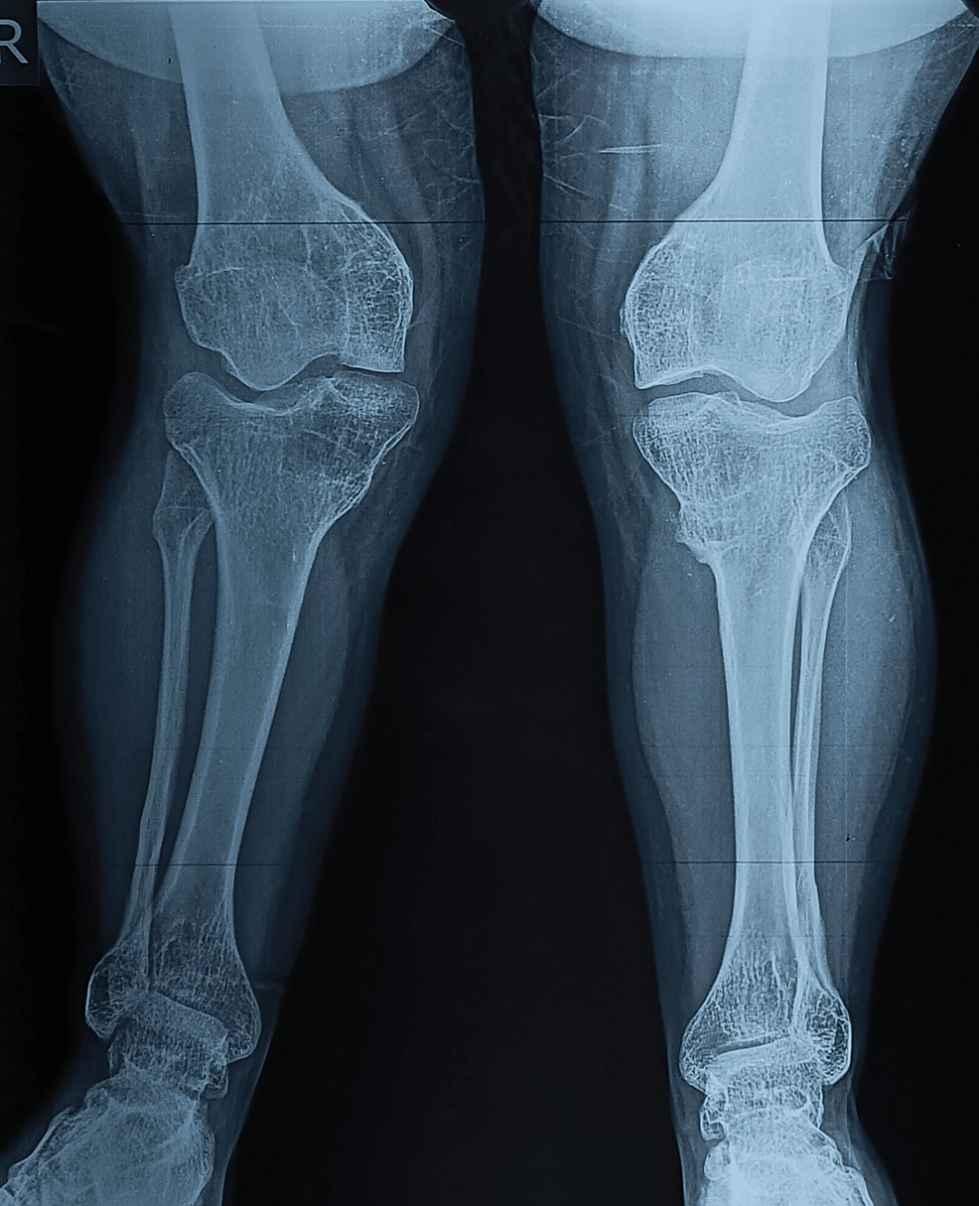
Radiographs at five-year follow-up showing good correction with no relapses.
